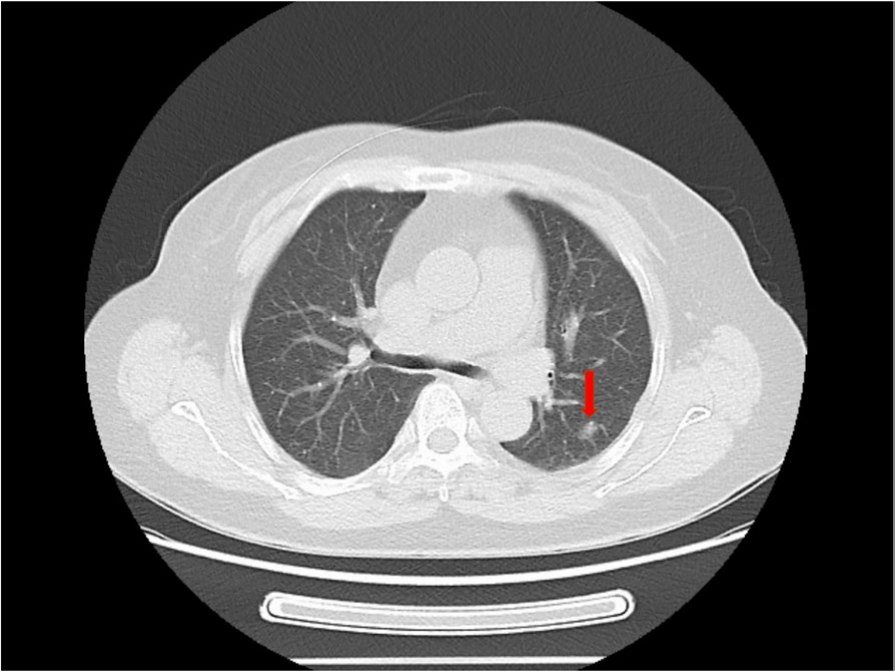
Chest computed tomography (CT) revealed a (1.2 × 0.8 cm), heterogeneous, hypodense nodule in the apico-posterior segment of the left upper lobe (red arrow, Fig. 1), demonstrating heterogeneous attenuation without calcification or cavitation
